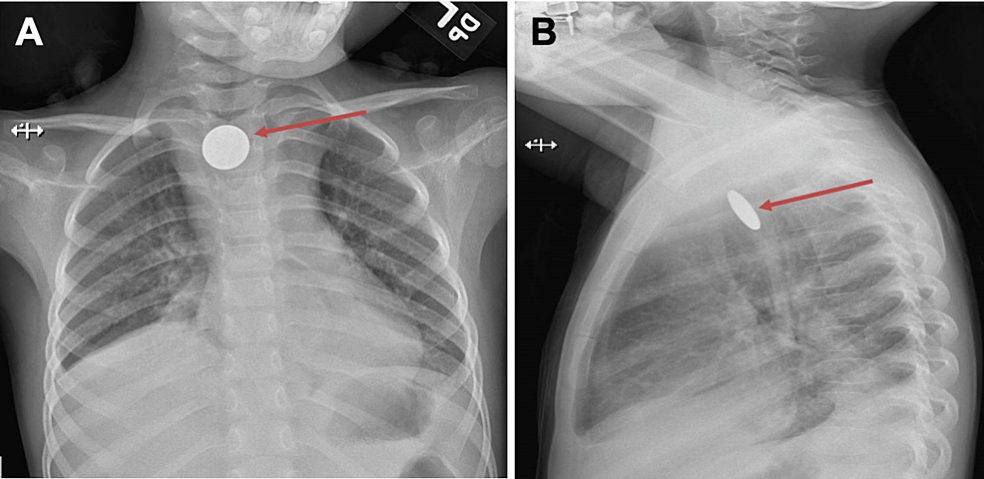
Chest X-ray anteroposterior (A) and lateral view (B) showing a radio-opaque shadow (red arrow) in the upper central thoracic region oriented in a coronal and tangential position, respectively.
